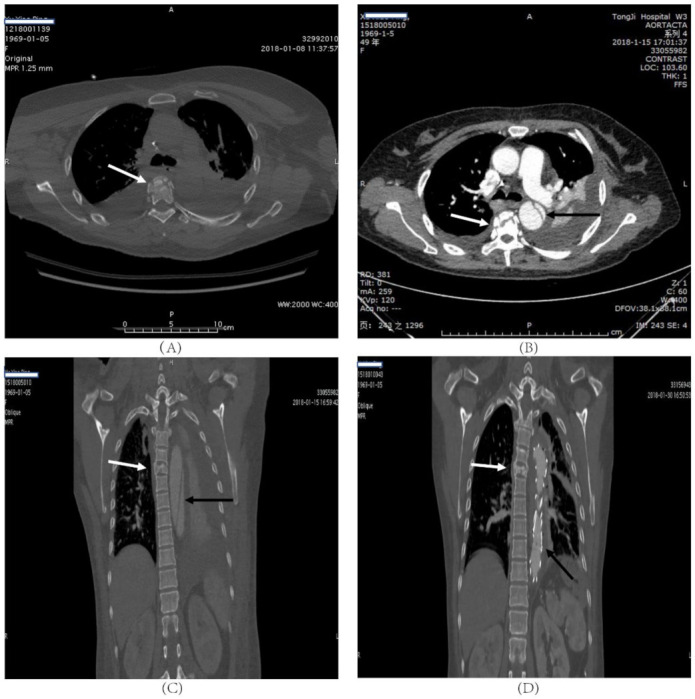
A forty-nine-year-old female who was involved in a chest compression injury. T5 fracture without paraplegia (ASIA D); (A) transverse CT scan showed thoracic vertebra fracture (white arrow), and the aortic injury was not obvious. (B) Transverse of CT angiography scan of the same patient showed that thoracic vertebra fracture (white arrow) and the aortic injury (black arrow). The aortic injury is obvious on the CT angiography scan. (C) Coronal reconstruction of the CT angiography of the same patient showing T5 fracture (white arrow) and formation of thoracic aortic dissection (black arrow). (D) Repeat CT of the same patient after the intervention, coronal reconstruction.
